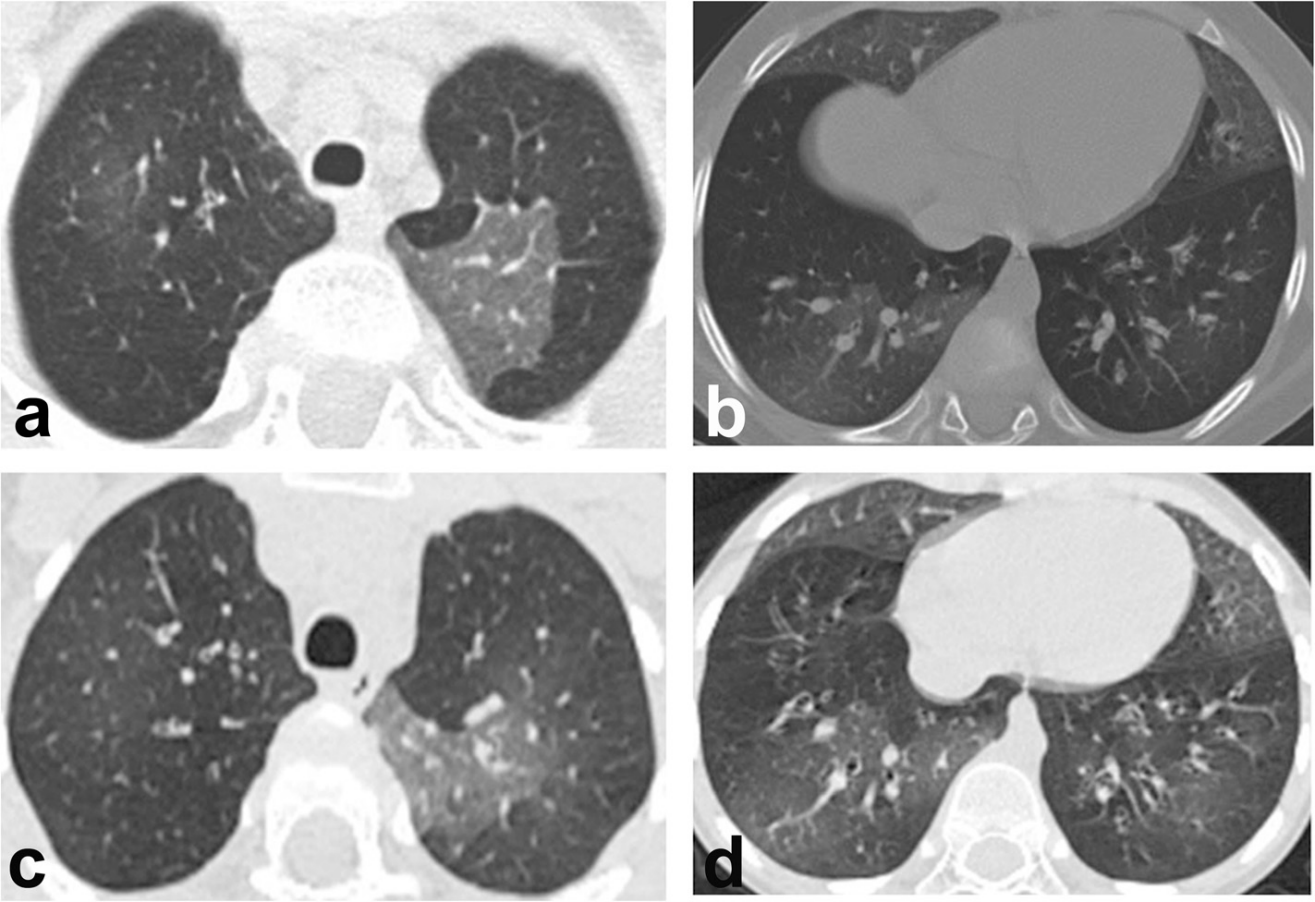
Baseline high-resolution computed tomography scans. Sharply defined areas of ground glass opacity more marked in the left upper lobe (a), in the middle lobe, lingula and right lower lobe (b). High-resolution computed tomography at the age of 6 years (after 3 years of oral hydroxychloroquine and azithromycin) showing unmodified ground glass attenuation in the same areas, indicating lung damage stabilization (c and d)
